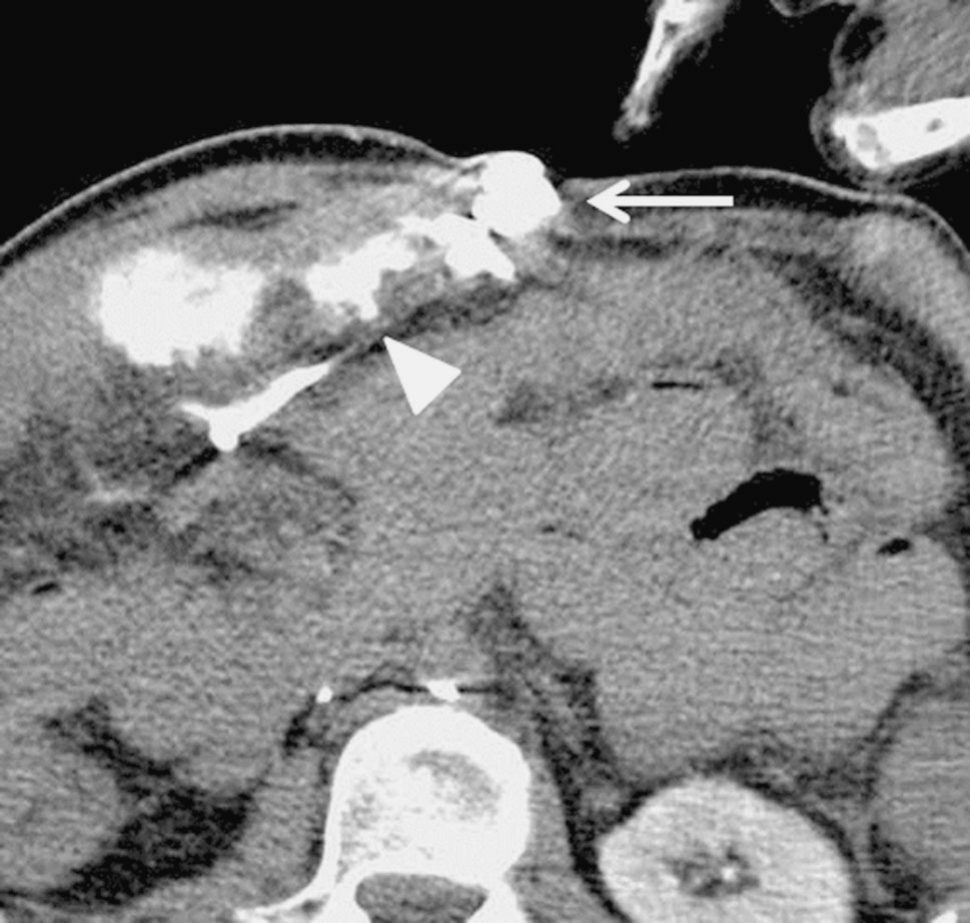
Retrograde CT images through the 18-gauge needle obtained by manually injecting contrast medium show mesenteric varices (*arrow*) and extravasation into the small bowel (*arrowhead*)
